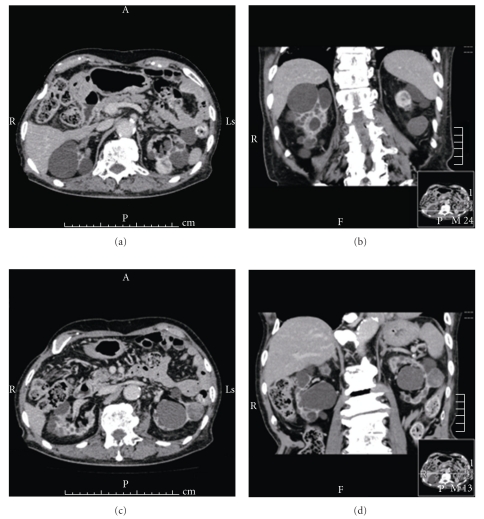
Abdominal computed tomography demonstrated two masses, 2.1 × 2.0 cm and 2.4 × 2.2 cm in diameter, at the middle of the left kidney with acquired cystic disease of the kidney.
